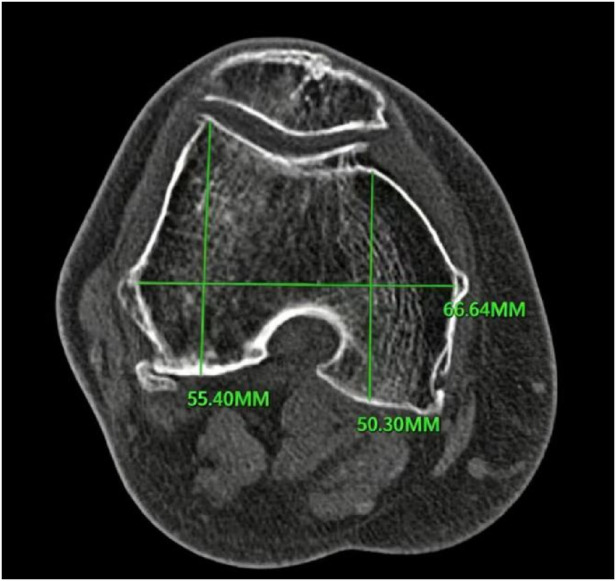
CT-based measurement of distal femoral morphology and aspect ratio. On the axial CT image at the level of the planned distal femoral resection, the mediolateral (ML) diameter was measured parallel to the surgical transepicondylar axis, and the anteroposterior (AP) diameter was measured perpendicular to the ML axis. An additional anteroposterior reference measurement was also recorded for morphological characterization. The aspect ratio was calculated as ML/AP.
